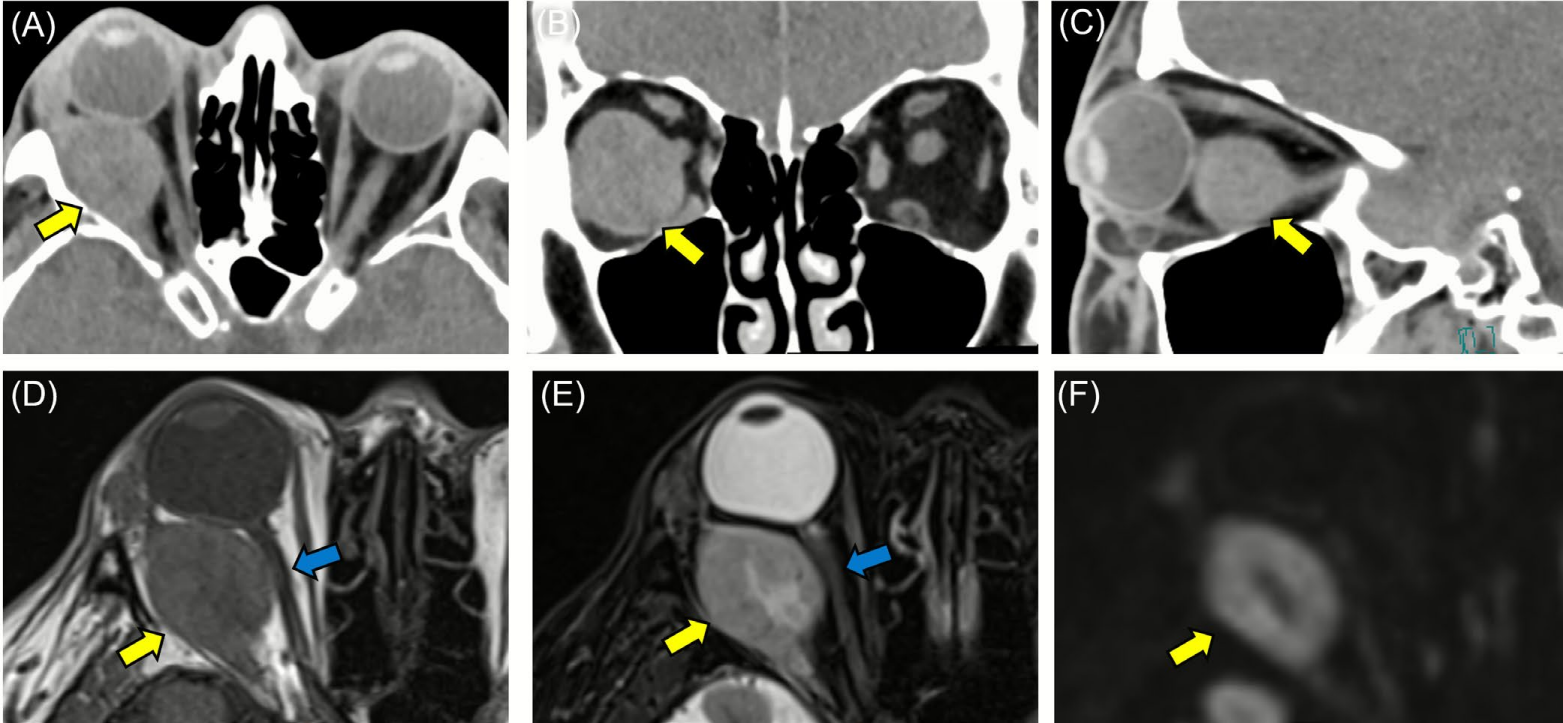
Imaging findings showing ocular adnexal metastasis from renal cell carcinoma. Head computed tomography scanning of the right orbital tumor lesion before treatment showed a round mass measuring 3.0 × 2.4 × 2.7 cm in the posterior‐lateral area of the right eyeball (A), axial section; (B), coronal section; (C), transverse section. This right orbital mass showed a slightly low signal level in magnetic resonance imaging T1 weighted images (WI) (D), a signal level similar to that of muscle tissue in T2WI (E), and a high signal level in diffusion weighted images (F). Yellow and blue arrows indicate the right orbital mass and right optic nerve, respectively.
